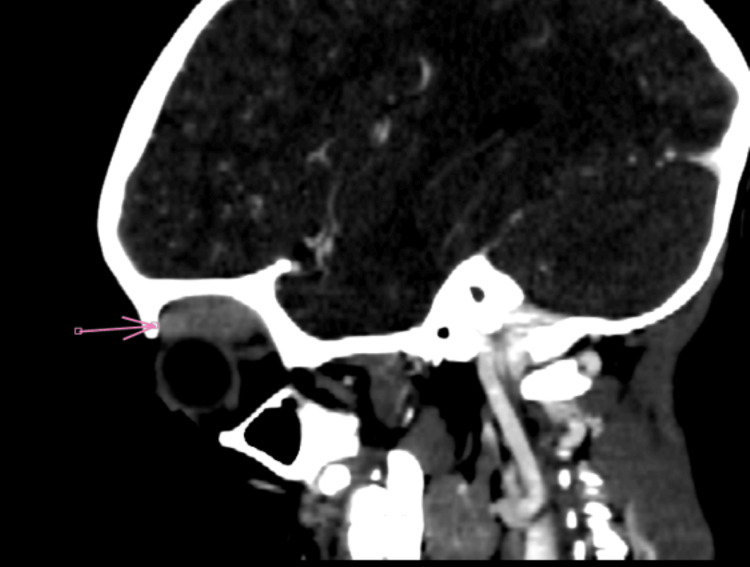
Sagittal non-contrast brain CT scan showing infiltration of the lacrimal gland with extraconal extension
